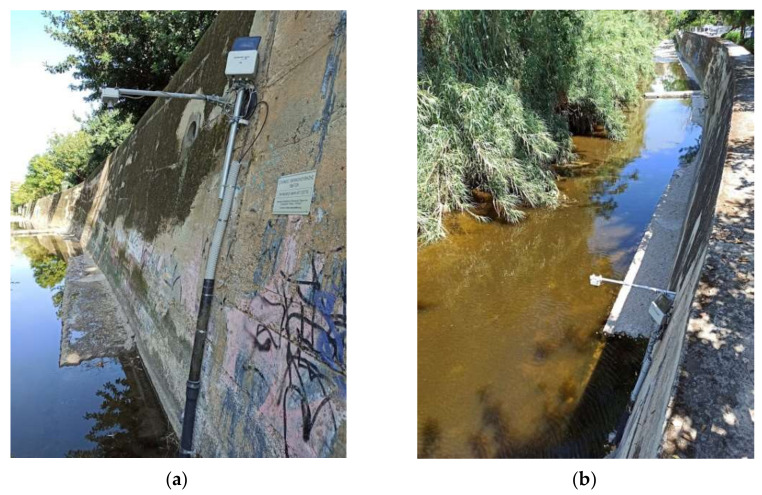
Two views (a,b) of the installation of the Hellenic Centre for Marine Research (HCMR) multiparameter sonde AT500 (including the pressure transducer) within the pipe attached to the wall and the ultrasonic sensor at the edge of the mounting bracket attached to the wall for water stage monitoring at the Pikordafni stream in the Attica region.
